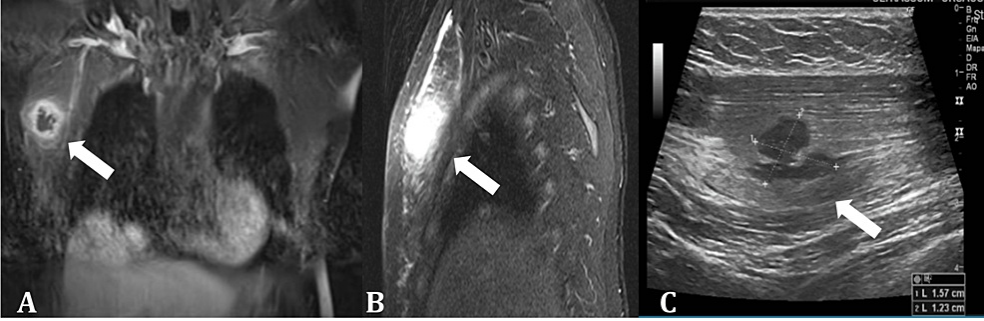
Magnetic resonance imaging of the thorax. A. T1W post-contrast coronal view, B. T2W post-contrast sagittal view, and C. Ultrasound gray scale, depicting the abscess at the right pectoralis major muscle (arrow).
